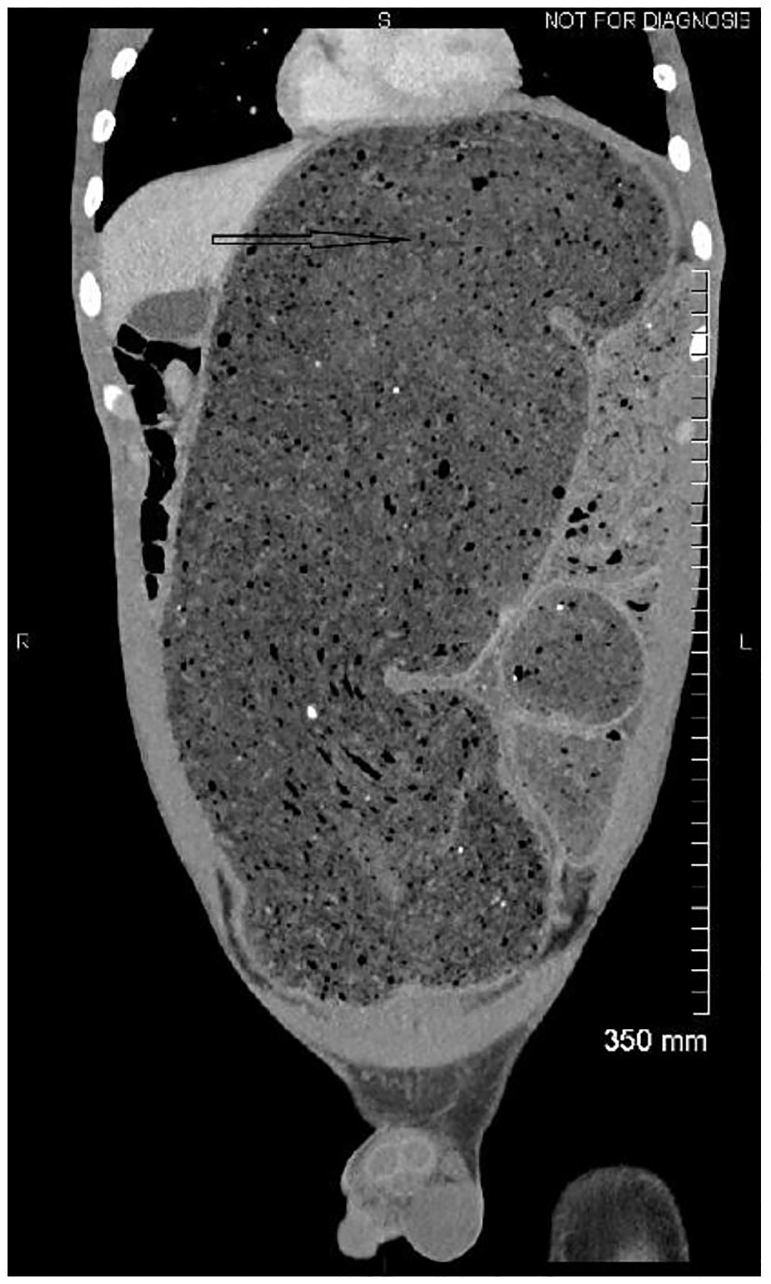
Coronal section of the abdomen by computed tomography displaying extent of the extensive stool burden.
